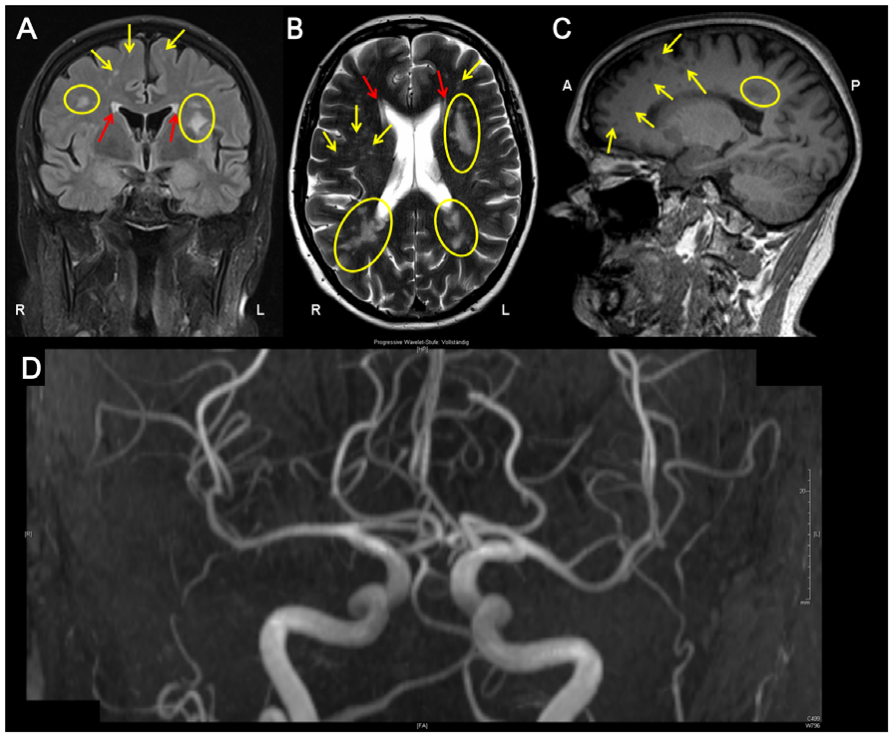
FLAIR- (A), T2- (B) and T1- (C) MR images of index patient II.7 showed widespread, punctuated (arrows) and confluent (yellow circles) WML from periventricular (yellow) to subcortical (red) without gadolinium enhancement. Lesions were associated with “black holes” in T1-weigthed images (C) as a surrogate of severe demyelination and axonal injury. MR-angiography (D) showed no signs of cerebral vasculitis or intracranial arteriosclerosis.
